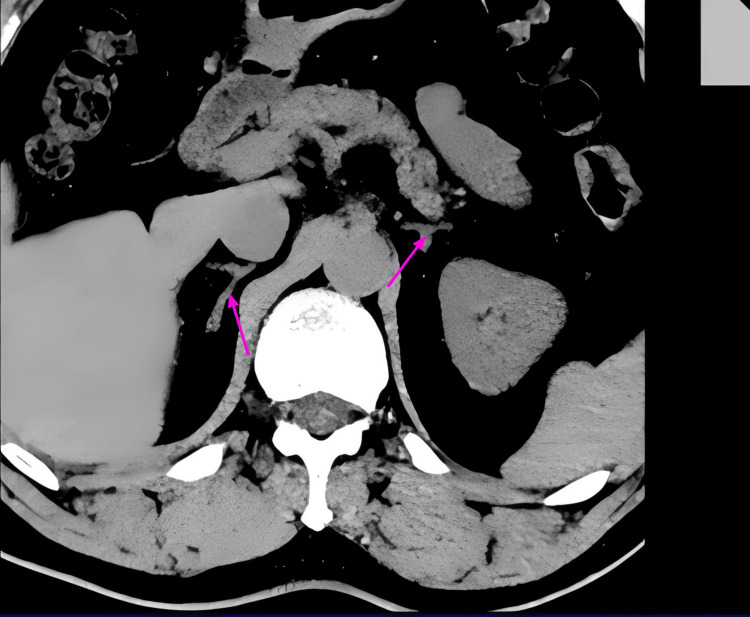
Non-contrast CT scan of the patient's father's adrenal glands
